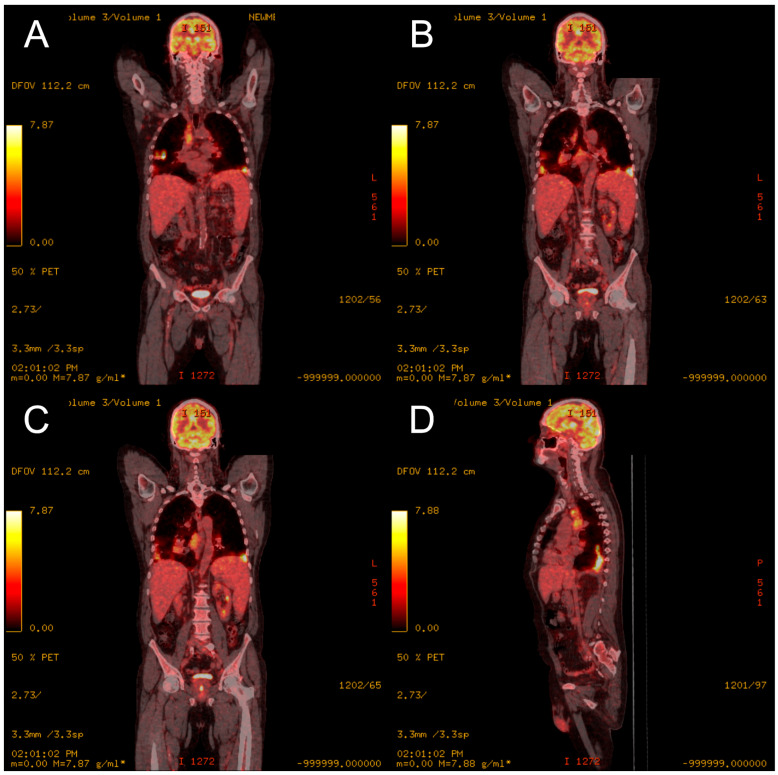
[¹⁸F]Fluorodeoxyglucose PET/CT from 2018 of the coronal plane (A–C) and sagittal plane (D), showing metabolically active mediastinal and hilar lymphadenopathy and pulmonary infiltrates.
